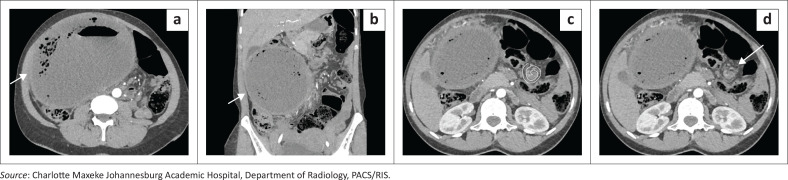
Splenic torsion in a 28-year-old female with known splenomegaly presenting with acute abdominal pain. (a, b) Axial and coronal contrast-enhanced CT images demonstrate absence of the spleen from its normal left upper quadrant location and the presence of an ectopic spleen within the lower abdomen (arrows). (c, d) The twisted vascular pedicle (“whirlpool sign”) is demonstrated (c and arrow in d). The spleen shows complete absence of enhancement with a central hypodense region containing internal air locules, consistent with infarction complicated by superimposed infection.
